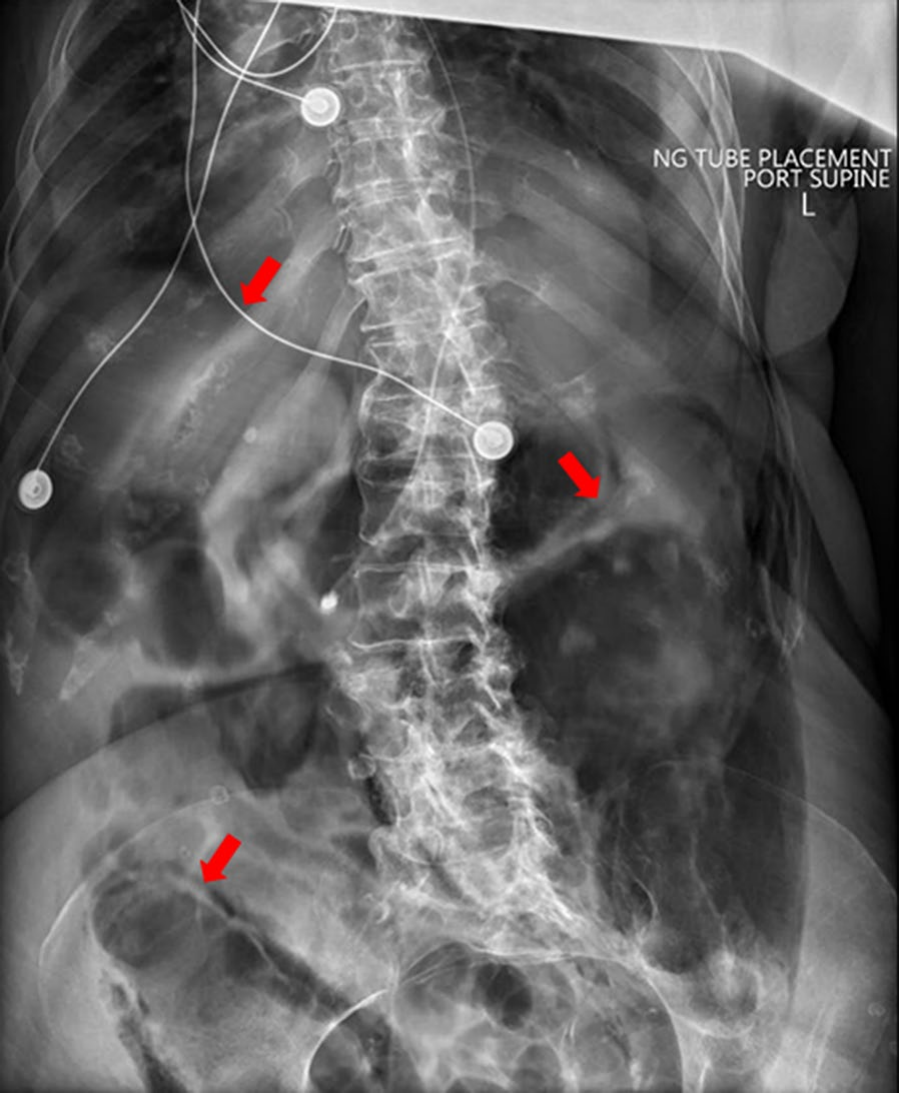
Abdominal X-ray showing free intraperitoneal air due to a small bowl perforation. This image depicts suprahepatic accumulation of free air, which is considered a hallmark sign of pneumoperitoneum
